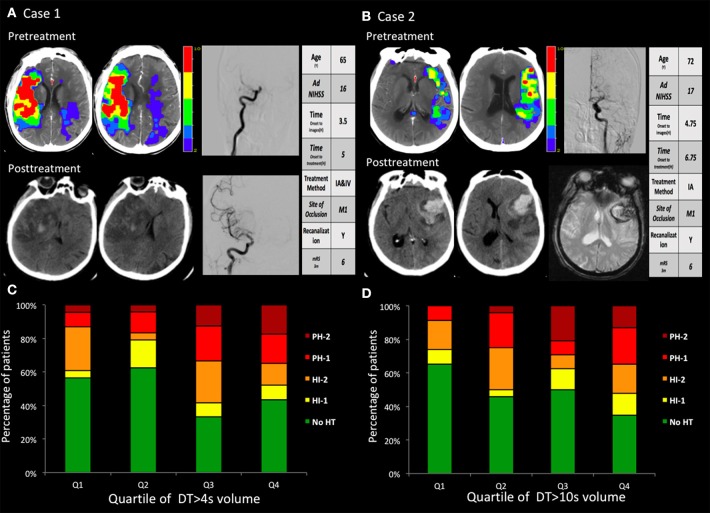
(A,B) two cases with hemorrhagic transformation. (A) Pretreatment CTP images show a marked large area with perfusion delay. The day after recanalization with intravenous and endovascular treatment, CT findings revealed a PH. The PH was located within the mildly and severely hypoperfused regions. (B) Pretreatment CTP images show small areas with large mild perfusion delay and small severe perfusion delay. After recanalization with endovascular treatment, CT and GRE findings revealed a large area of PH. The PH was located within the mildly hypoperfused regions. (C,D) The volume in the regions with baseline mild and severe perfusion delay (DT >4 s and DT >10 s) correlated with the PH. Q1 represents the patients with the lowest quartile volume, whereas Q4 indicates patients with the highest quartile volume.
